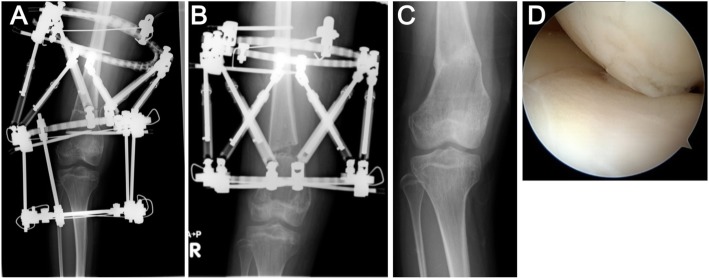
Postoperative anteroposterior radiographs and arthroscopic images of the patient by TSF. a Immediately after femoral osteotomy. b Gradual correction was achieved over the course of 18 days. c Four months later, bone union was confirmed and the external fixator was removed. d Arthroscopic findings showing an intact articular surface
